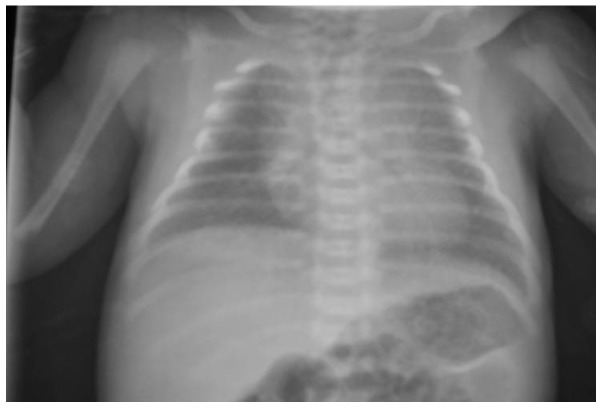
Frontal chest radiograph of the patient aged 1 day revealed hyperinflation, diffuse opacification and air bronchogram of the lungs.
